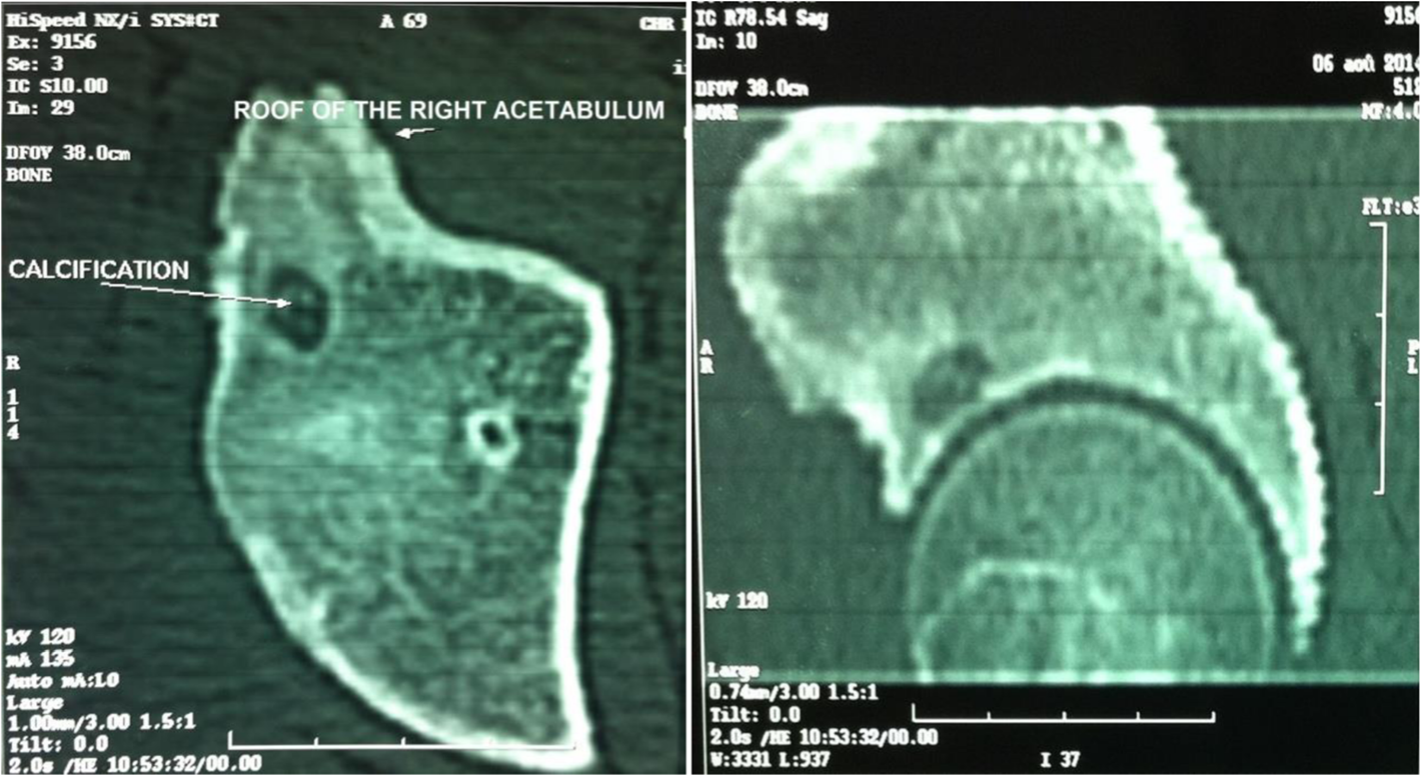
Computed tomography scan showing an osteoid osteoma at the acetabular roof
